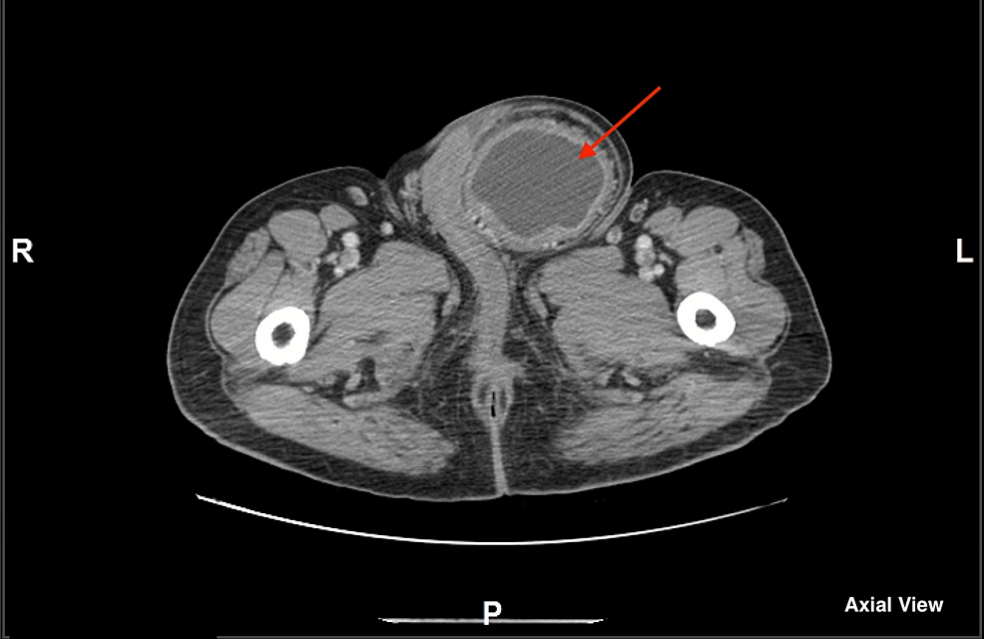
CT Abdomen and Pelvis with contrast (Axial View) Large left inguinal hernia containing a significant part of the bladder (red arrow).
